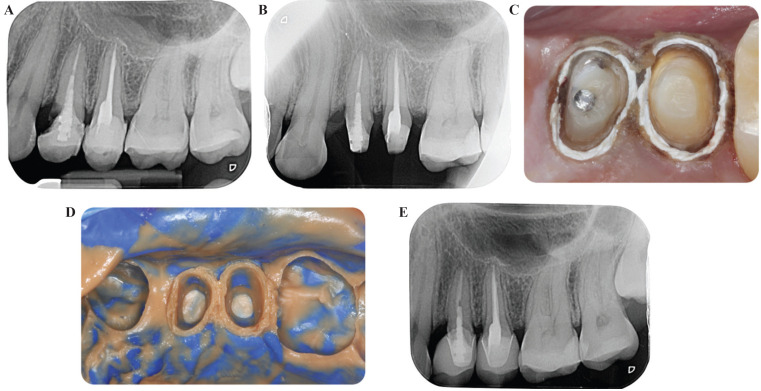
(A) Preoperative periapical radiograph. (B) Periapical radiograph of the reconstruction and preparations. (C) PTFE tape placed into the sulcus. (D) Impression of the preparations. (E) 1 year follow up of the definitive crowns.
